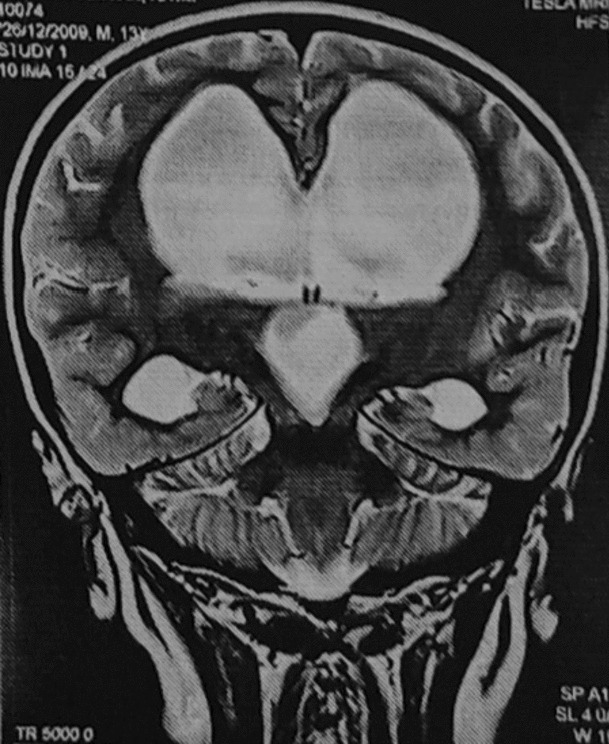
T2-weighted coronal MRI of brain showing dilated lateral and third ventricle
